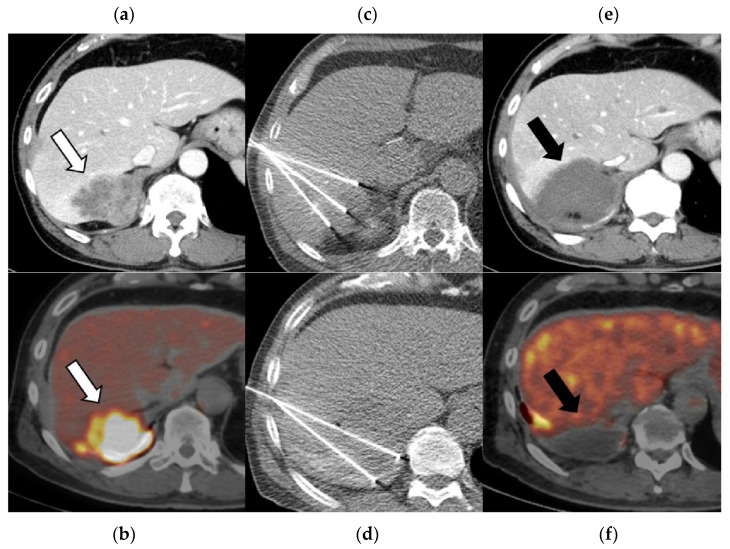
Images of 64-year-old man with sarcomatoid pleural mesothelioma. Baseline axial CT (a) and 18F-FDG PET/CT (b) images showing a large recurrent tumor invading the liver (white arrow). The patient underwent chemotherapy and immune checkpoint inhibitor therapy; however, the tumor continued to enlarge. Radiofrequency ablation (RFA) was subsequently performed in three sessions (c,d), resulting in successful tumor ablation. Tumor enhancement on contrast-enhanced CT and avid FDG uptake on PET/CT (black arrows) were no longer observed on follow-up CT (e) and PET/CT (f).
